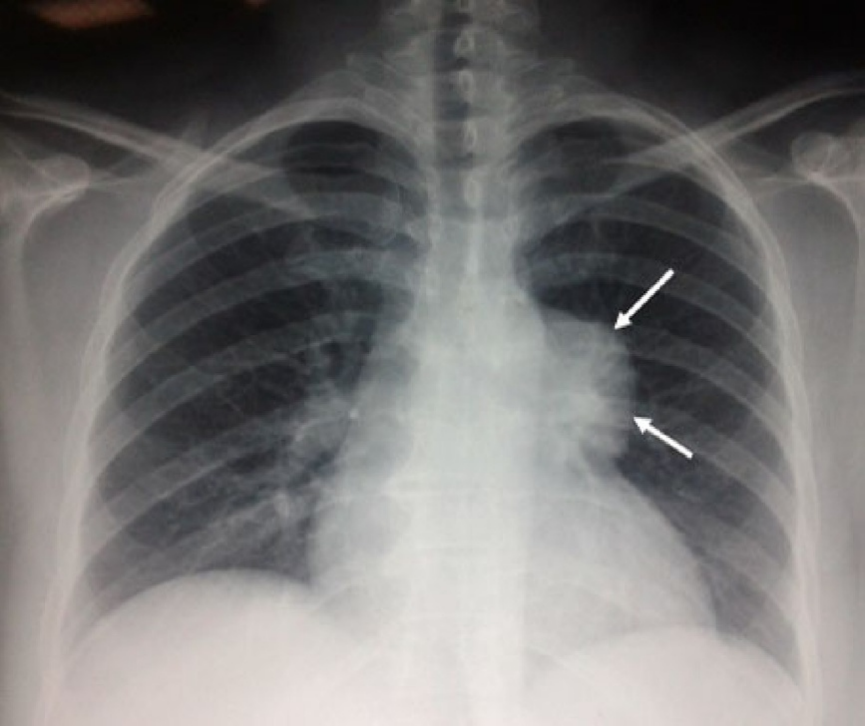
Chest x-ray showing a large well defined opacity in the left hilar region.
